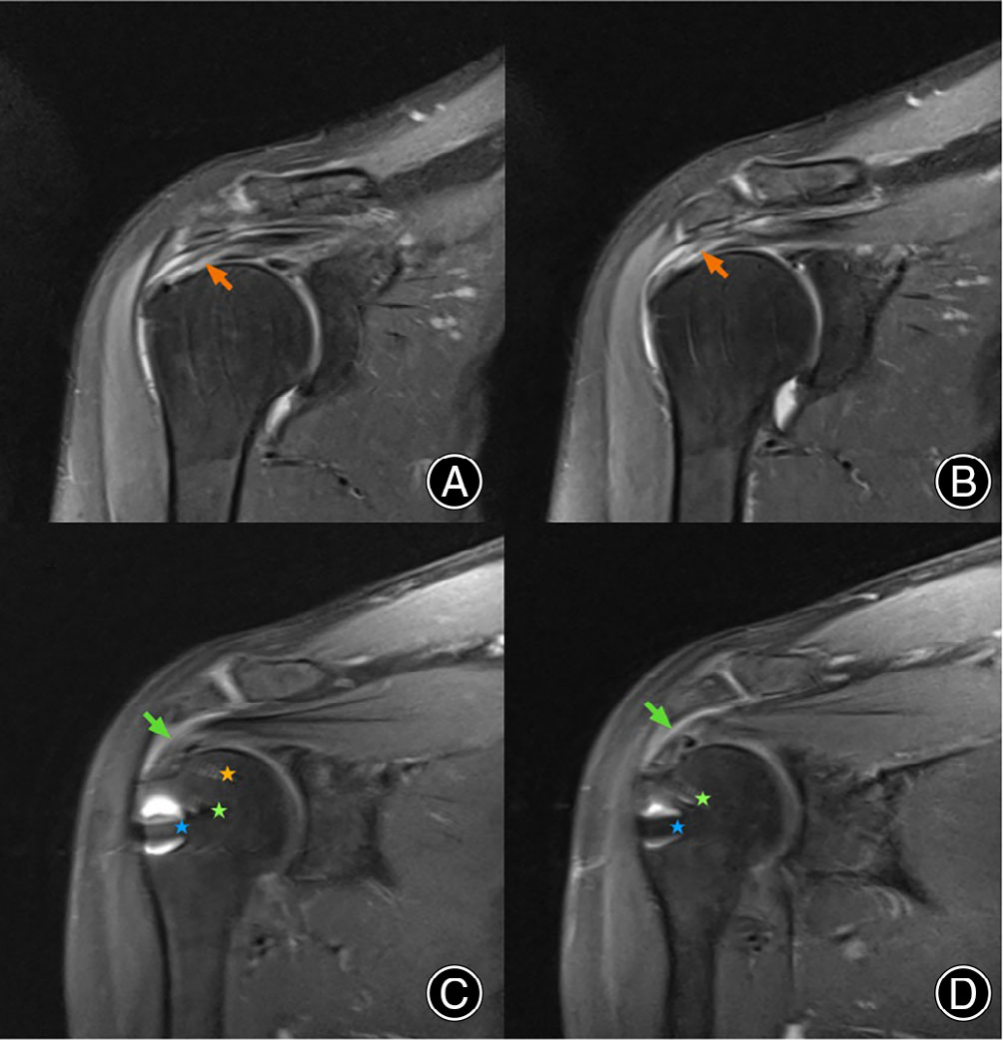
This figure presents preoperative and postoperative MRI (T2 coronal view) images following the application of the modified arthroscopic triple‐row repair technique for an L‐shaped delaminated rotator cuff tear. Images A and B are preoperative MRIs highlighting the delamination of the rotator cuff, indicated by orange arrows. Images C and D represent MRI scans taken six months postoperatively, where green arrows point to significant healing at the bone interface of the rotator cuff and the greater tuberosity footprint area, as well as the disappearance of delamination. The orange star marks the location of the medial‐row anchor, the green star indicates the middle‐row anchor, and the blue star identifies the lateral‐row anchor. It is noted that the high signal area surrounding the lateral‐row anchor is due to artifact produced by the metal sleeve within the anchor.
